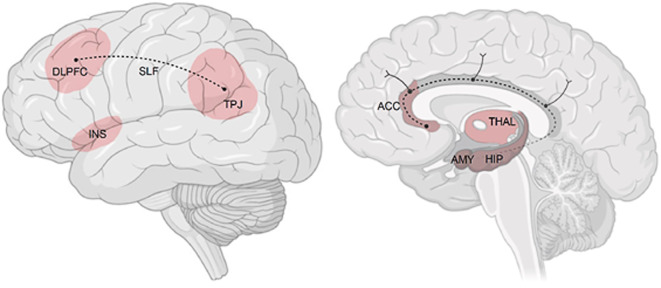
Brain networks associated with (1) affective disorder (AD) in cross-sectional structural and functional magnetic resonance imaging studies; (2) disease trajectories in AD; and (3) cognitive–emotional mechanisms (emotion regulation, expectation, social cognition, and cognitive–behavioural rhythms) hypothesized to interact with periods of symptom change. *DLPFC* dorsolateral prefrontal cortex, *INS* (anterior) insula, *TPJ* temporoparietal junction, *ACC* anterior cingulate cortex, *THAL* thalamus, *AMY* amygdala, *HIP* hippocampus, *SLF* superior longitudinal fasciculus. (© T. Kircher, F. Stein)
